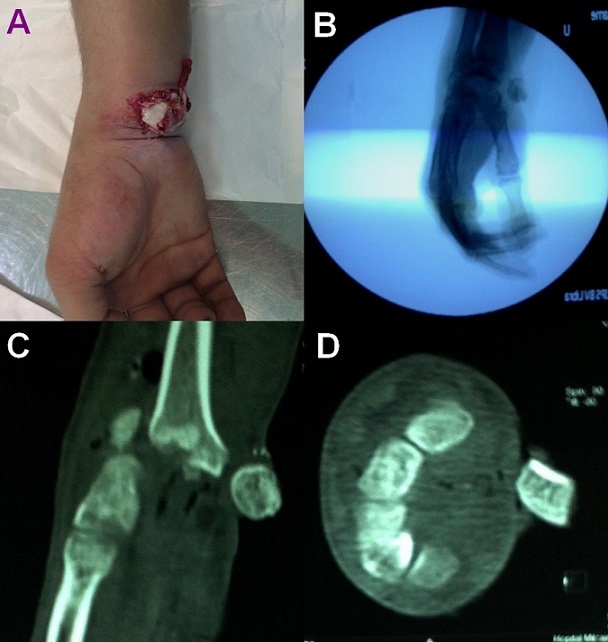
(A) clinical aspect at admission revealing an open enucleation of a carpal bone, (B) X-ray of the left wrist shows a lunate dislocation, (C and D) computed tomography scan (transverse and sagittal cut) shows lunate enucleation with scaphoid and radial styloid fracture
